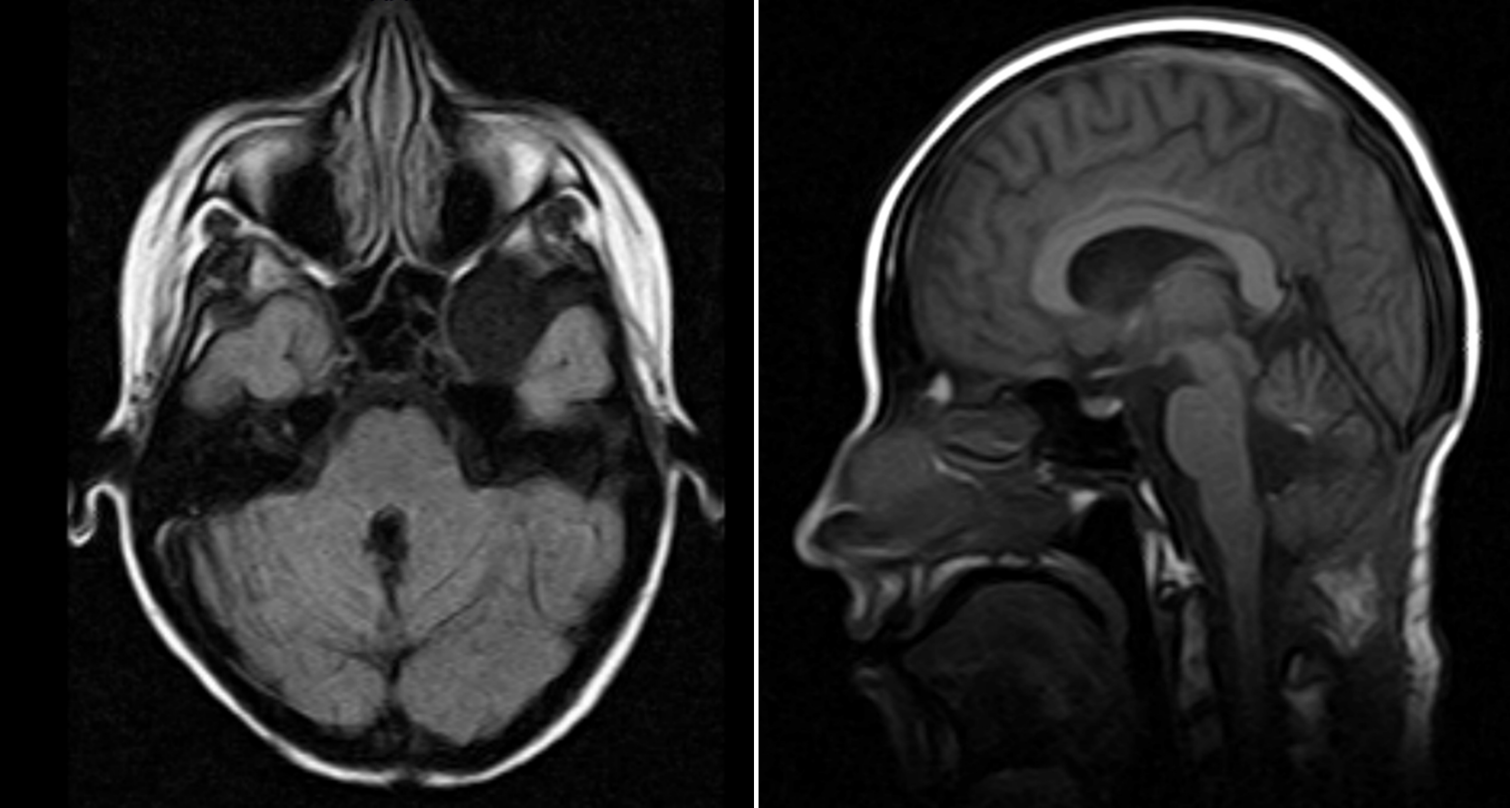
Postoperative magnetic resonance imaging (MRI) in February 2011 Left: Axial view Right: Sagittal view
